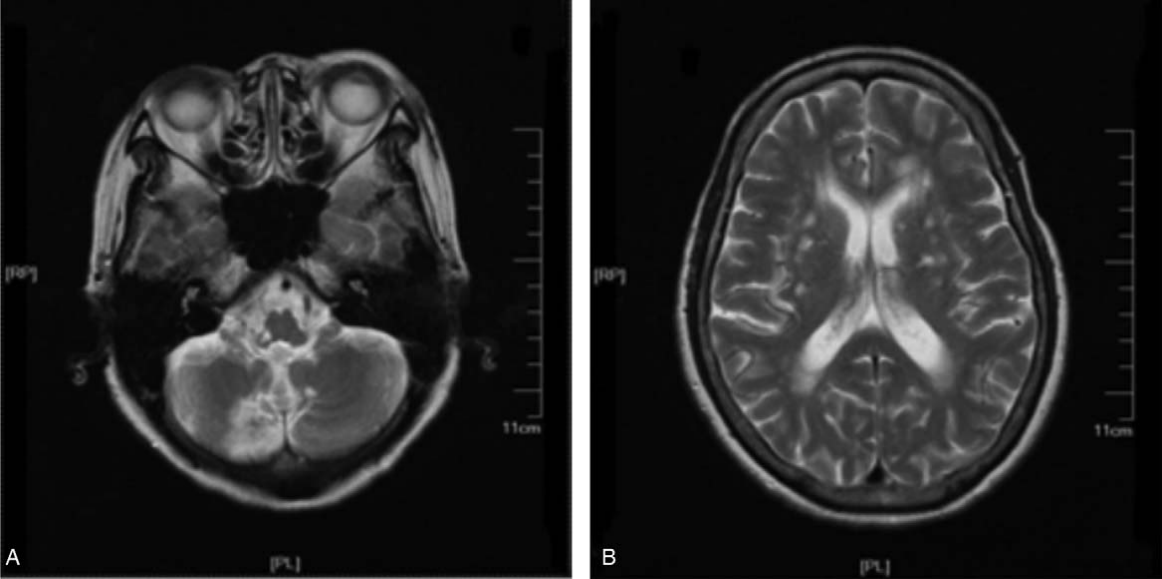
Brain MRI of the case showed patchy acute infarction in the right medulla oblongata and the right cerebellar hemisphere (A), multiple small patches of ischemia, infarction, and softening lesion also occurred in the pons, beneath the bilateral frontoparietal cortex, centrum semiovale, usually at the side of the lateral ventricle and in the basal ganglia (B). MRI = magnetic resonance imaging.
